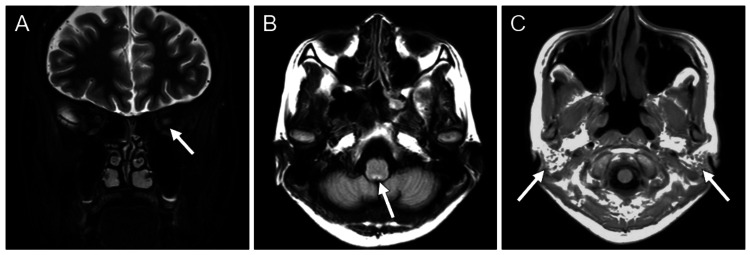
Brain magnetic resonance imaging on the fifth day of hospitalization. (A) Coronal short tau inversion recovery image shows enlargement and hyperintensity of the left optic nerve. (B) Axial fluid attenuated inversion recovery image reveals a hyperintense area in the area postrema. (C) Axial T1-weighted image shows atrophy, fatty infiltration, and punctate soft tissue signals in the parotid gland, reflecting Sjogren's syndrome.
